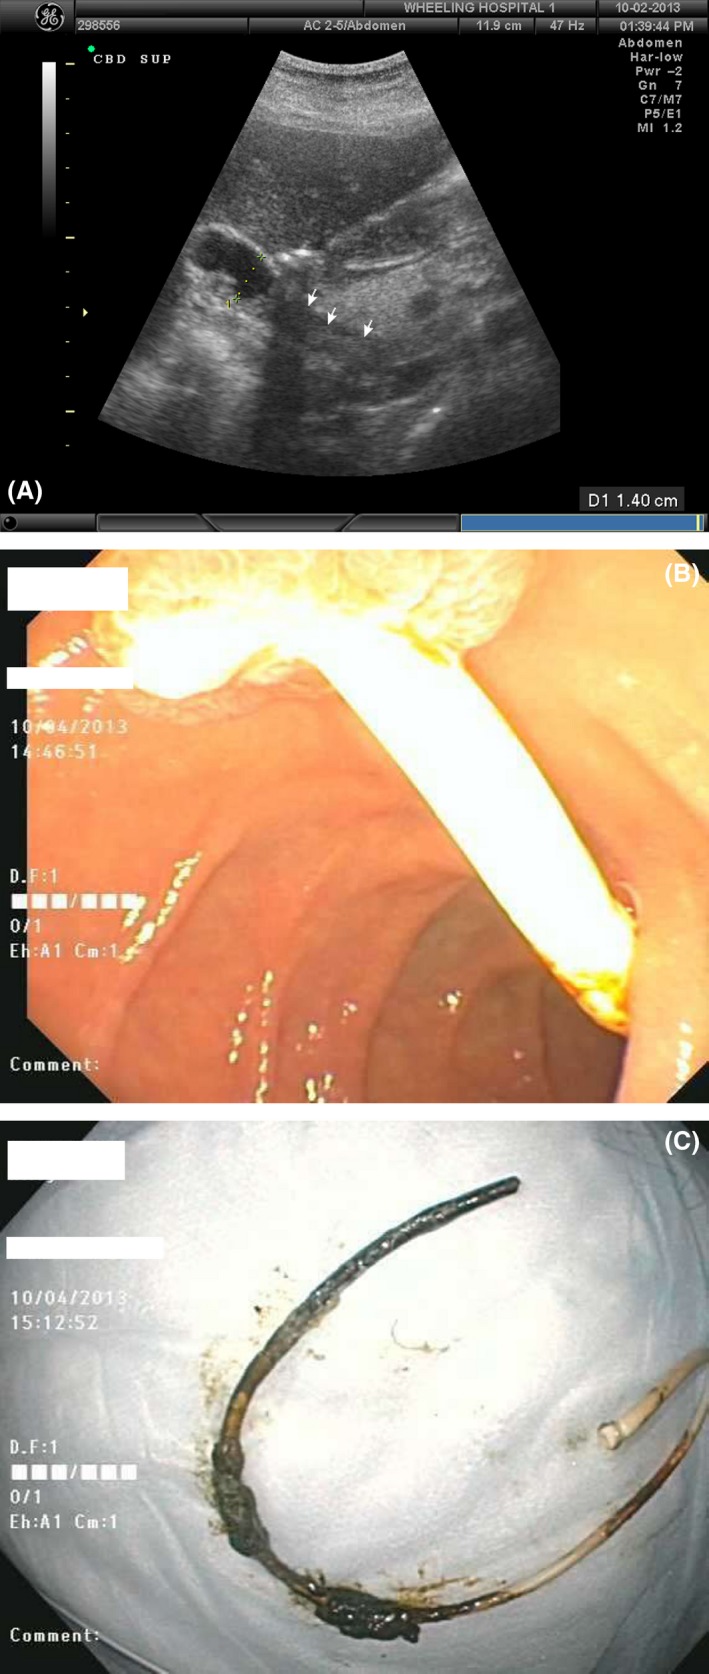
(A) Ultrasound showing a foreign object retained within the common bile duct. (B) ERCP image demonstrating retained shunt lodged within the ampulla. (C) Long segment of retained ventriculo‐gallbladder shunt following sphincterotomy and endoscopic retrieval.
